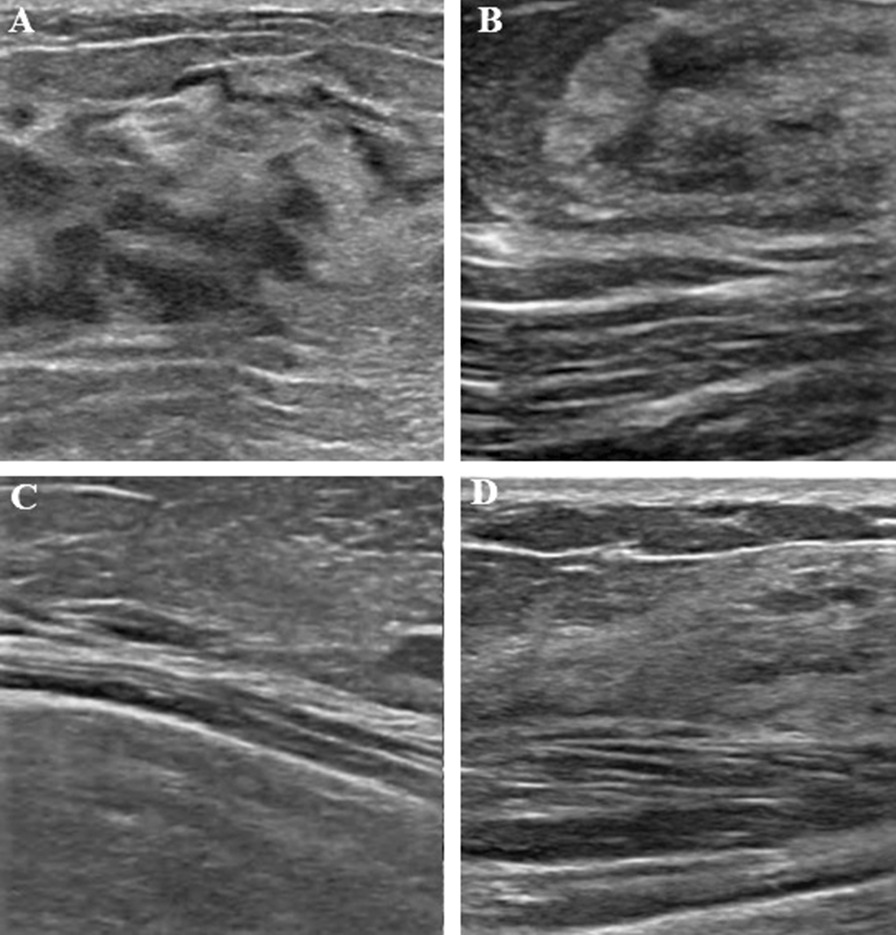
Ultrasonographic classification. a heterogeneous category (high percentage of fatty tissues), b ductal category (the inner diameters of ducts > 50% of the thick mass of the breast), c Mixed category (the inner diameters of ducts was 50% of the thick mass of the breast), and d Fibrous category (a dense classification of the breast)
